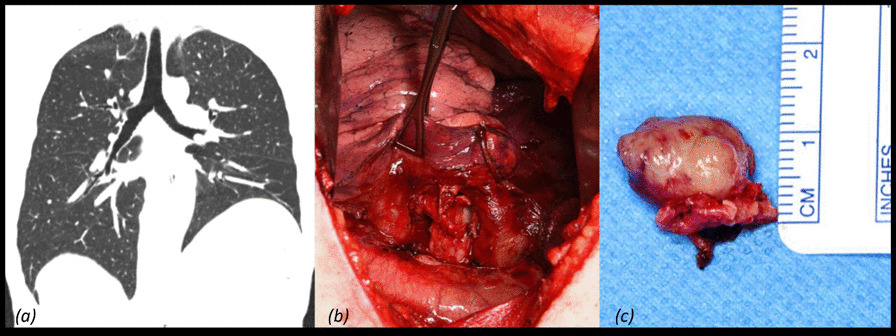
(a) CT chest of patient with typical carcinoid tumour in the left main bronchus, (b) bronchectomy in the same patient, (c) resected tumour.
